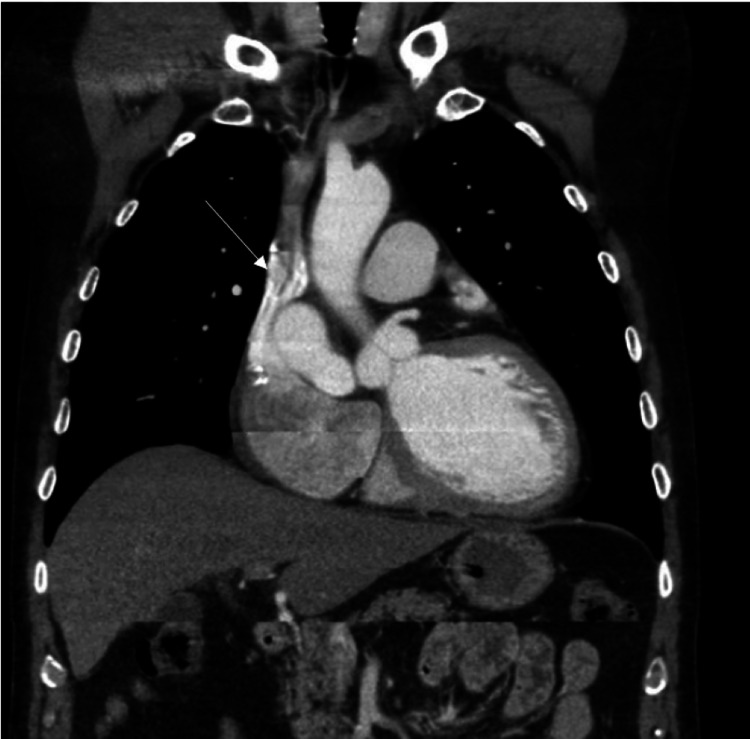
Chest CT showing saccular fistula (white arrow) measuring 3.6 x 3.3 cm connecting the right coronary artery to the right atrium.
